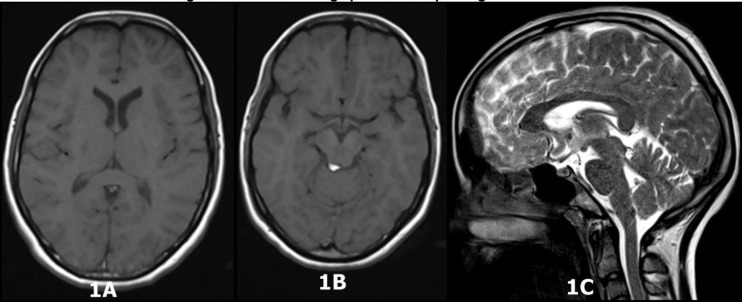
MRI Brain showing lipoma in the quardrigeminal area
